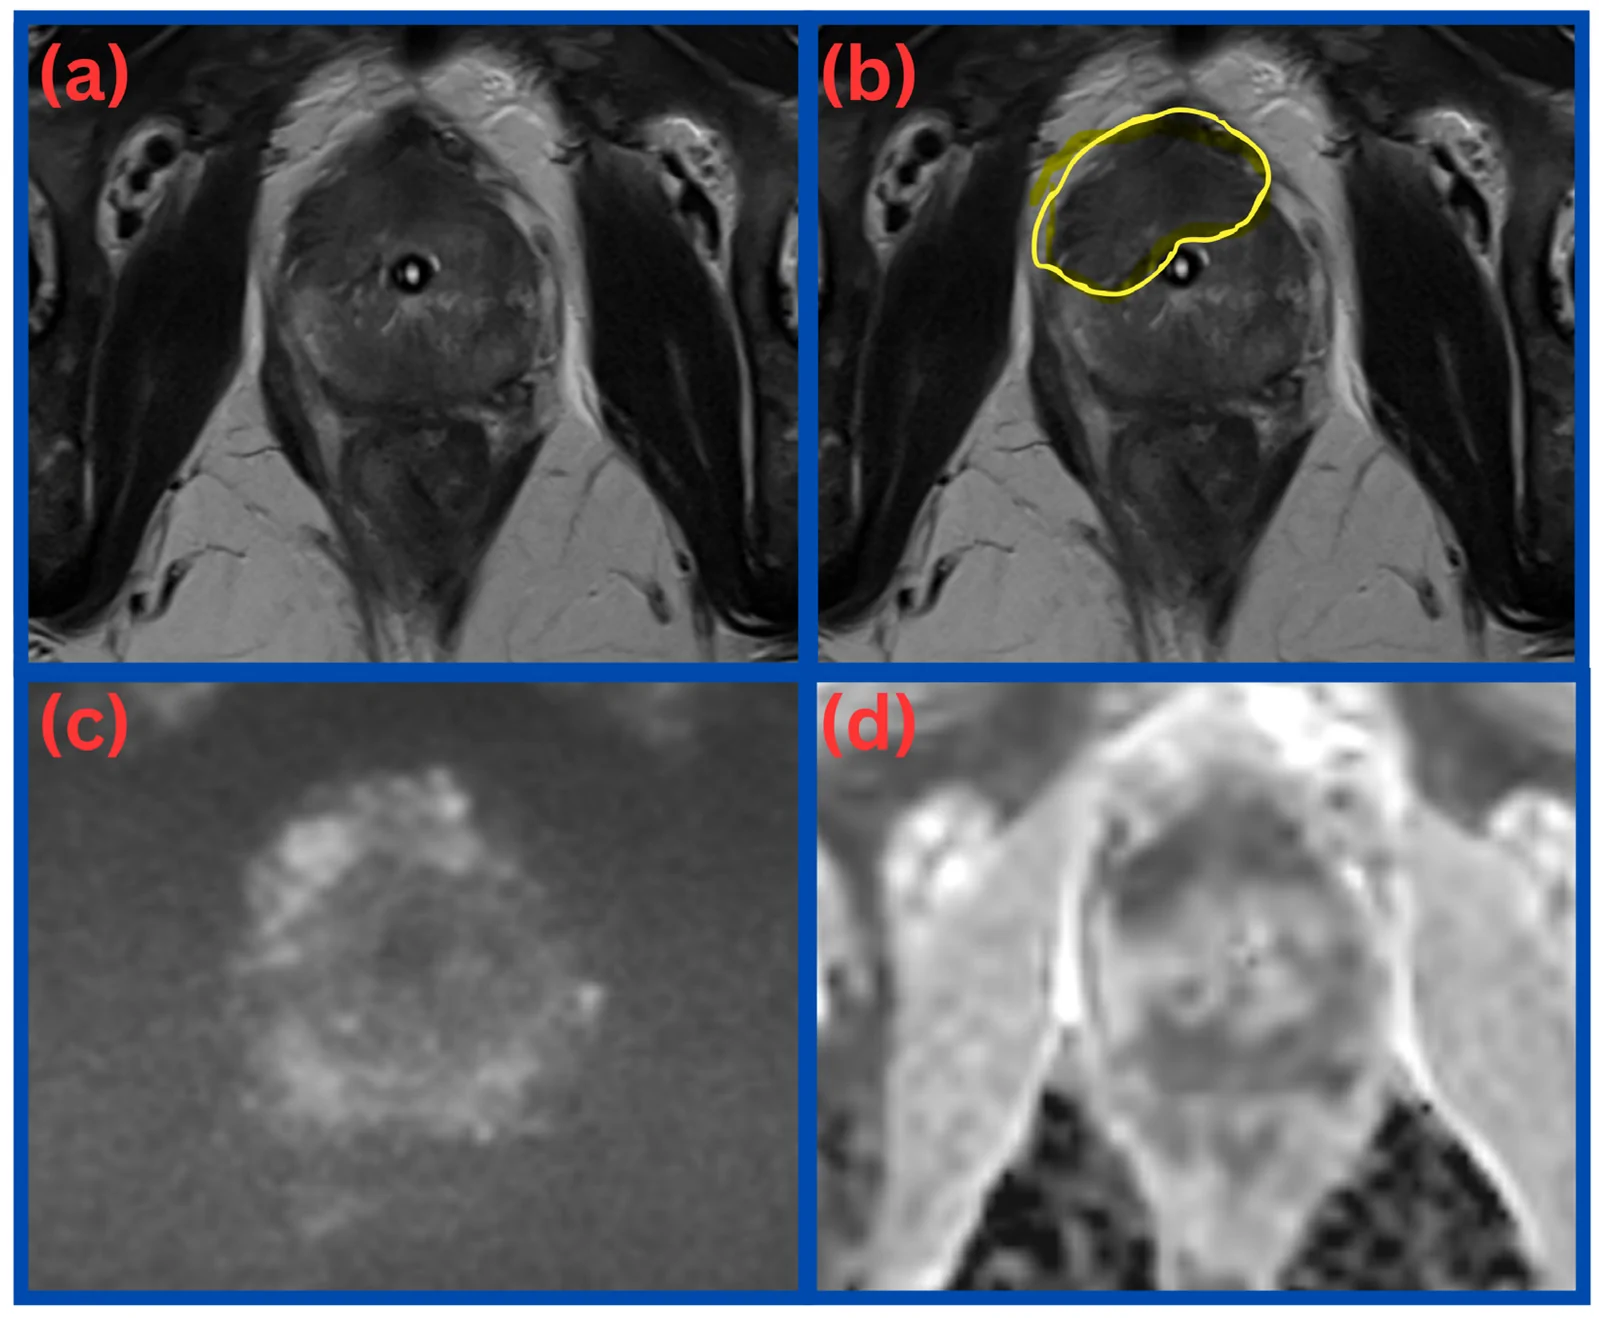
Multiparametric MRI of a PI-RADS 5 lesion with extraprostatic extension. (a) Axial T2-weighted image showing a diffusely marginated area of T2 hypointensity in the anterior portion of the transition zone that appears to infiltrate and extend beyond the prostatic capsule, and (b) the corresponding axial T2-weighted image with the lesion outlined in yellow. (c) High *b*-value diffusion-weighted image (*b* = 1400 s/mm^2^) shows marked high signal, and (d) the ADC map shows marked corresponding hypointensity, indicating pronounced diffusion restriction. A lesion of this size and signal characteristics with apparent capsular breach corresponds to a PI-RADS 5 (clinically significant cancer highly likely) assessment. The anterior transition-zone location exemplifies the disease phenotype that transrectal systematic sampling reaches least reliably; Section 3.2 details the supporting quantitative evidence, including a per-patient relative risk of 2.55 (95% CI 1.56–4.16) and an odds ratio of 2.67 (95% CI 1.42–5.00) favoring transperineal detection of anterior, transition-, and central-zone disease, together with a concordant non-significant trend for anterior lesions in the PERFECT randomized trial.
